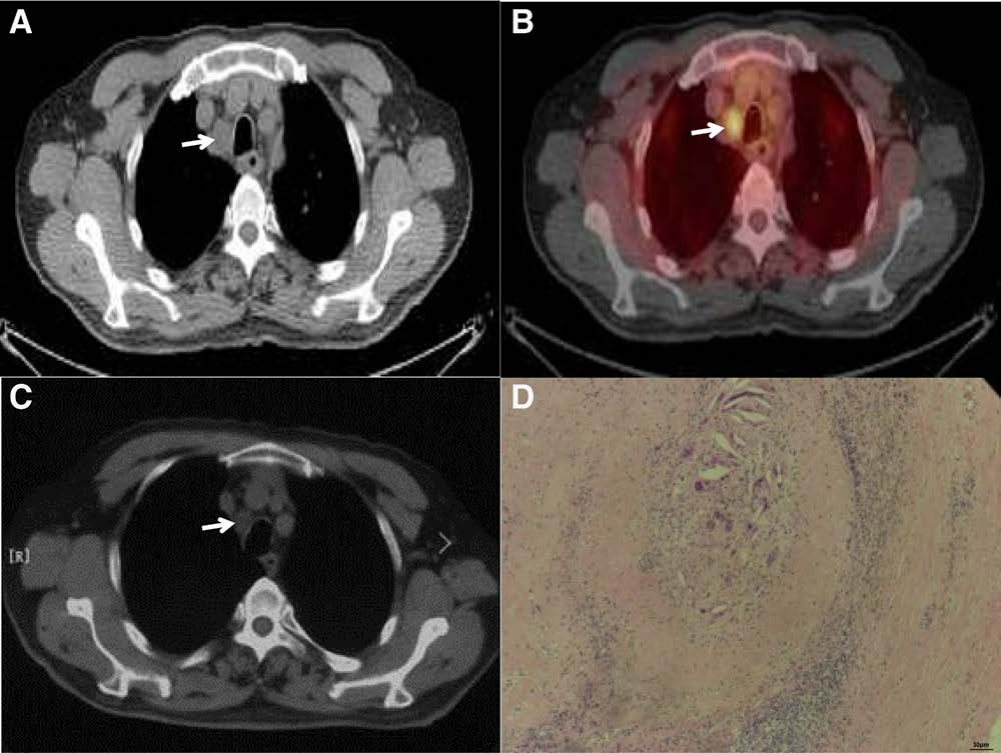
Efficacy evaluation of NCT for metastatic lymph nodes of ESCC. (A) CT confirmed that the lymph nodes adjacent to the right recurrent laryngeal nerve were metastatic lymph nodes (short diameter: 1.7 cm), (B) PET/CT confirmed that this lymph node was a metastatic lymph node, (C) right recurrent laryngeal parastinal lymph node reduction after chemotherapy (short diameter: 1.0 cm), and (D) TRG confirmed that the metastatic lymph nodes has achieved a pCR. ESCC = esophageal squamous cell carcinoma, NCT = neoadjuvant chemotherapy, pCR = pathological complete response, TRG = tumor regression grade.
